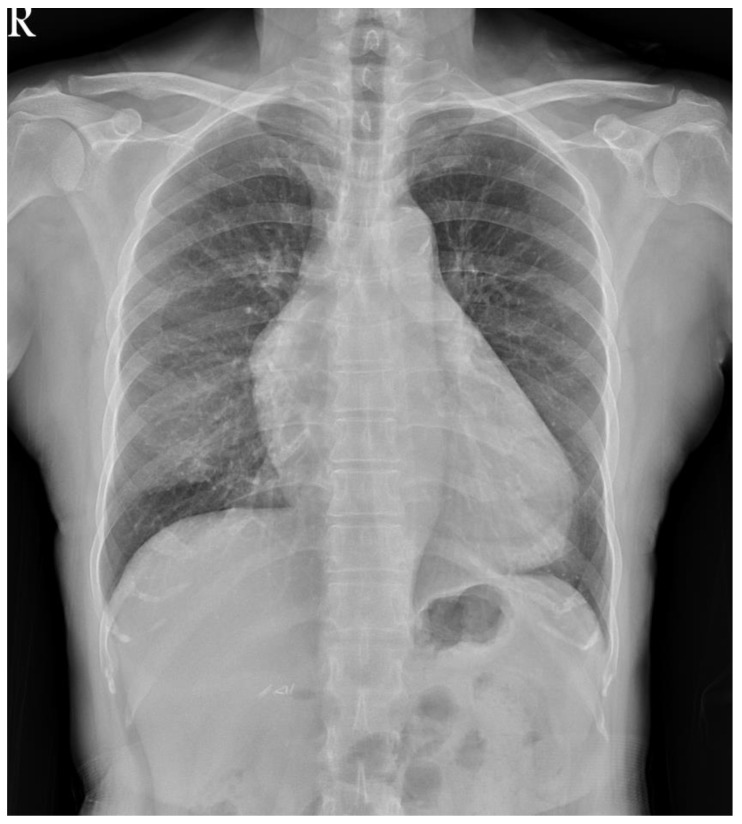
Chest radiography revealed increased soft tissue opacity over the mediastinum.
